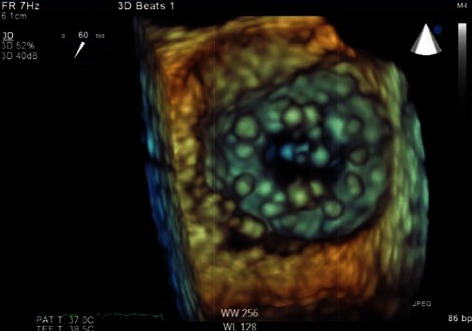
3D transesophageal echocardiogram of the normally functioning mechanical mitral valve at 60 degrees following treatment with tPA.
